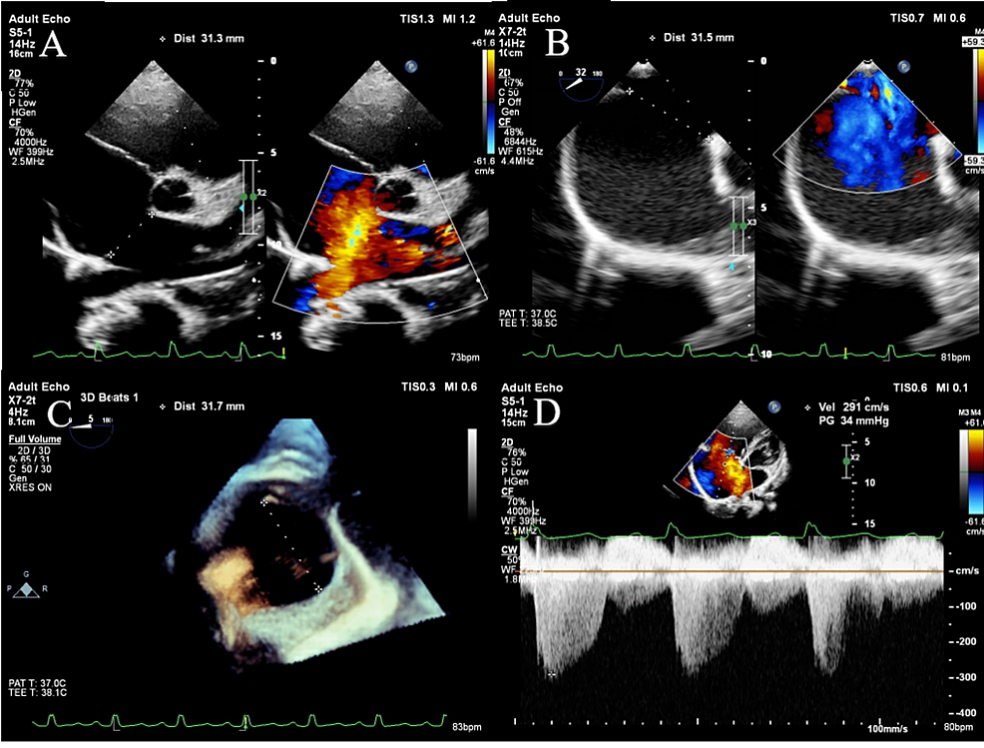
1A: ASD size on TTE; 1B: ASD size on TEE ; 1C: ASD size on 3D TEE; 1D Pulmonary hypertension ASD: Atrial Septal Defect; TEE: Transesophageal Echocardiography
